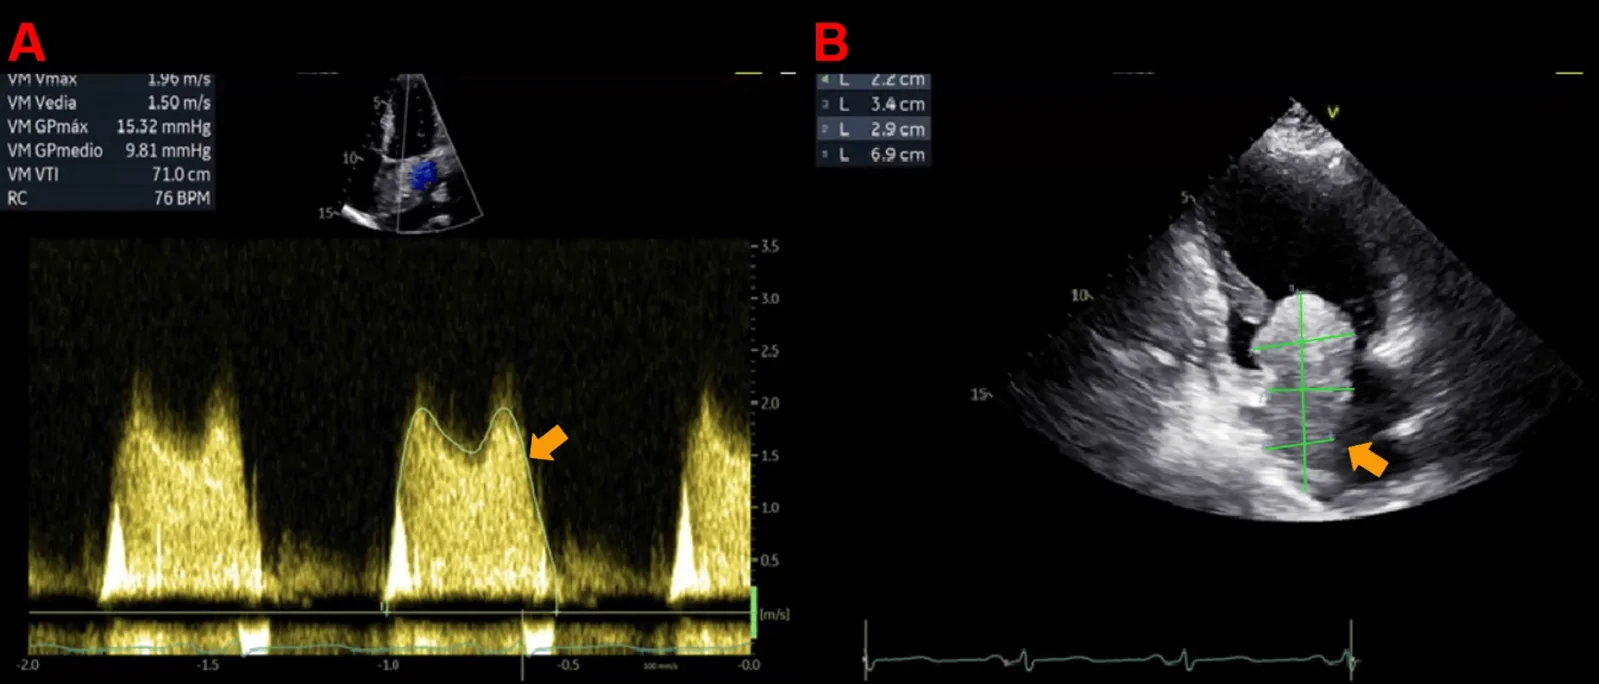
Echocardiographic characterization of the left atrial mass (A) Continuous-wave Doppler across the mitral valve demonstrating dynamic transmitral inflow obstruction caused by a "ball-valve" mechanism due to diastolic prolapse of the left atrial mass (orange arrow), generating a mean transmitral pressure gradient of 9.81 mmHg, consistent with hemodynamically significant functional mitral stenosis. (B) Apical four-chamber view demonstrating a large left atrial mass attached to the interatrial septum (orange arrow). Multiplanar measurements show maximum dimensions of 69 × 34 × 31 mm, consistent with a giant left atrial myxoma.
